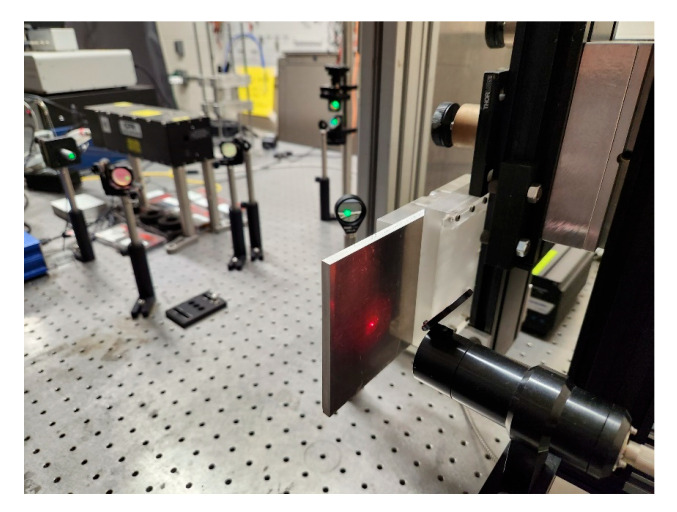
Measuring the impulse responses of a sample plate using a Laser Ultrasonic Test (LUT) system in B-scan mode.
